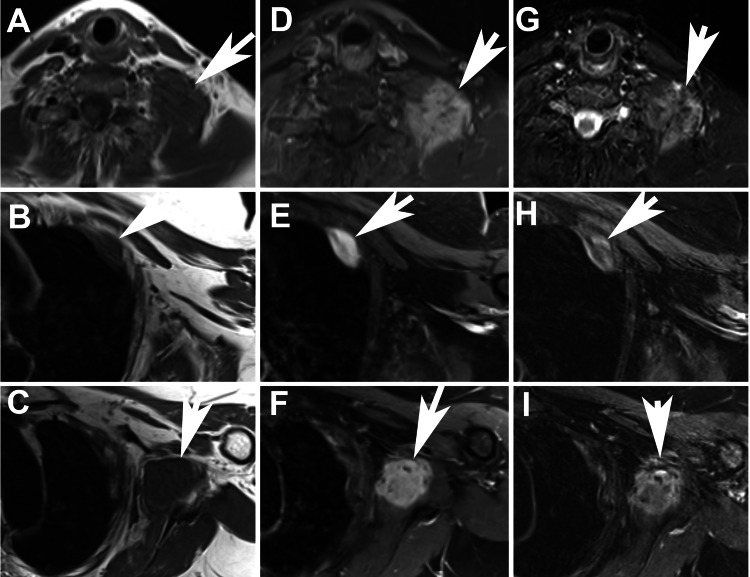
Axial MR images of Case 3 Axial MR images of Case 3. Top row (A, D, G): neck lesion; middle row (B, E, H): chest wall lesion; bottom row (C, F, I), subscapularis lesion. Left column (A-C): T1 sequence; middle column (D-F): T1 fat saturation sequence, post contrast; right column (G-H): STIR sequence. Arrows indicate tumor STIR: short tau inversion recovery
